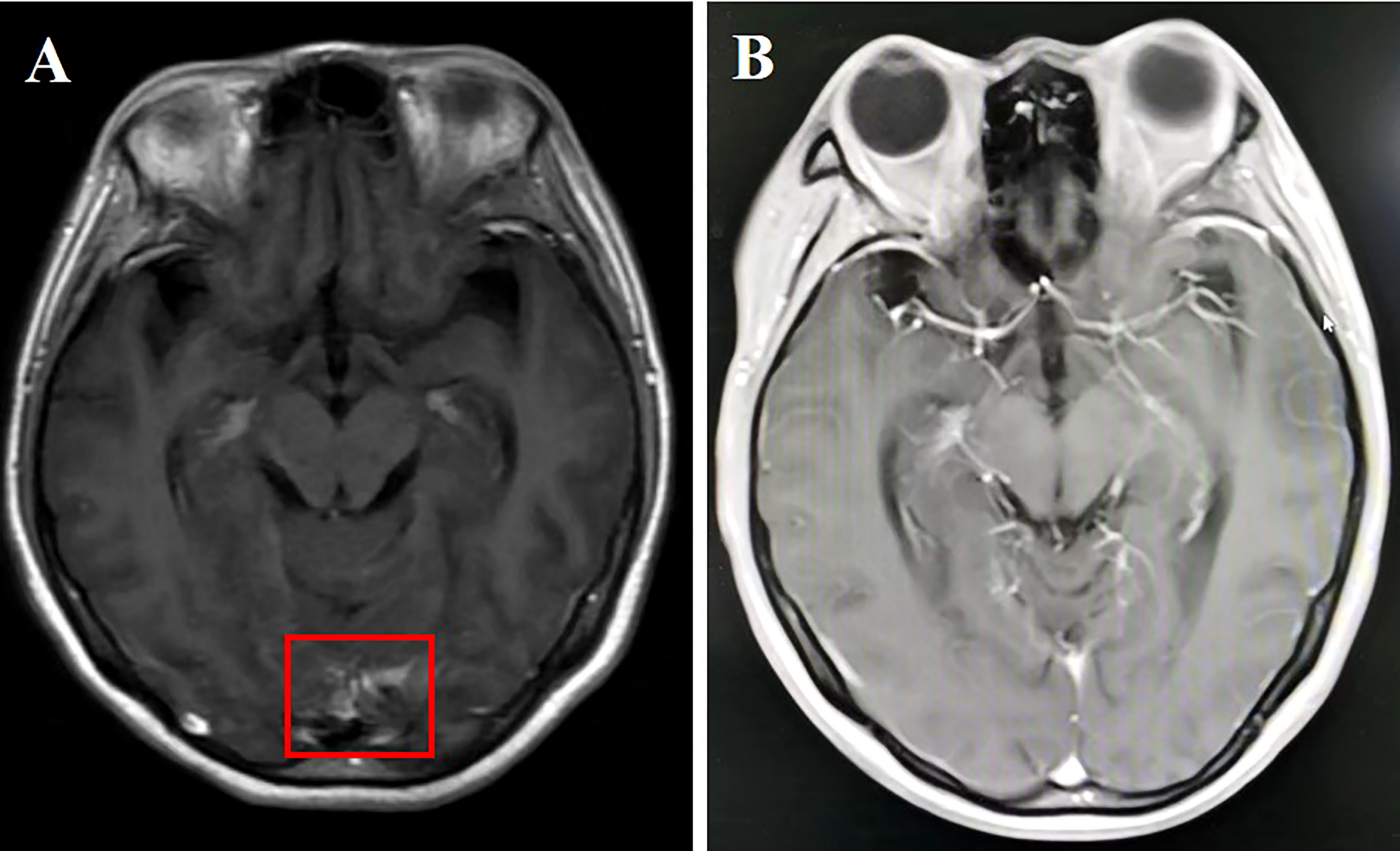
(A) Cranial enhanced MRI showing linear enhancement of leptomeninges in the temporal lobe (red box); (B) linear enhancement of leptomeninges disappeared 2 months after aumolertinib plus bevacizumab treatment.
